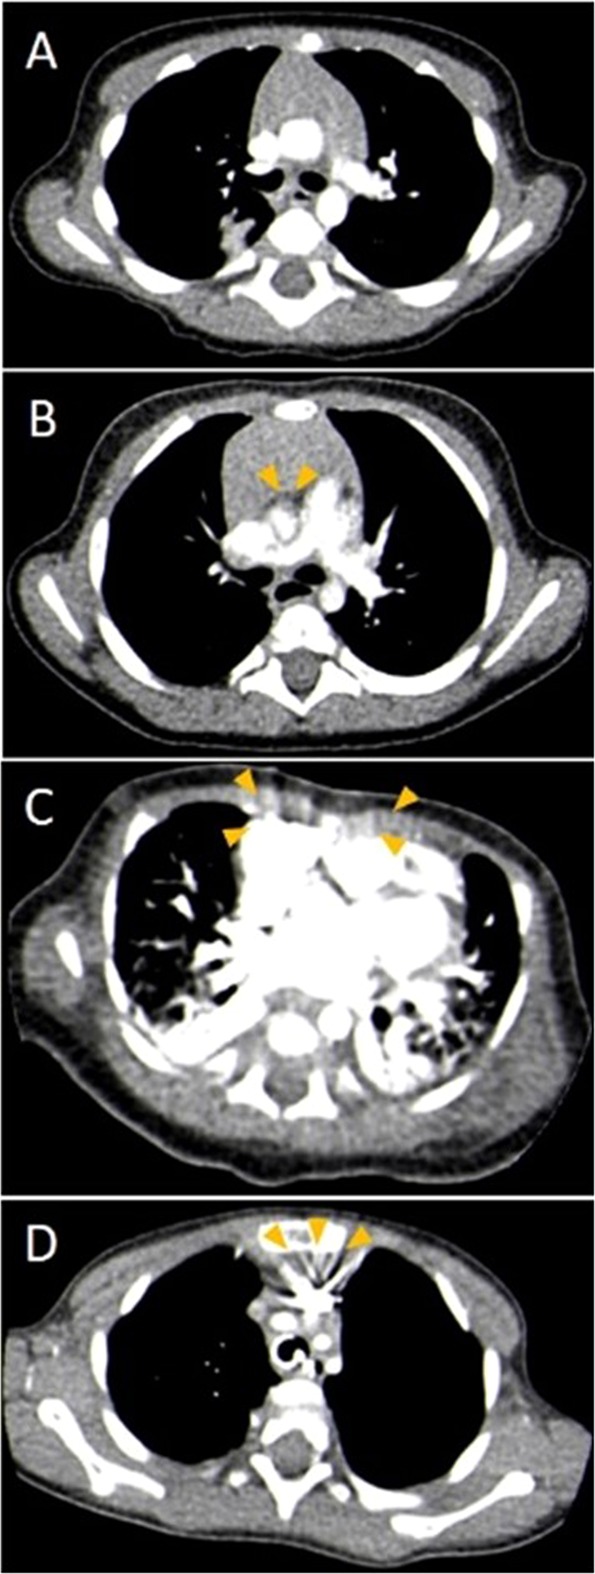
Representative axial CTA images in standard mediastinal window settings (width 400 HU, center 40 HU) to demonstrate different image artefact categories in subjective image analysis (A) no artefacts, (B) minor artefacts, (C) major artefacts, (D) extensive image blurring.
